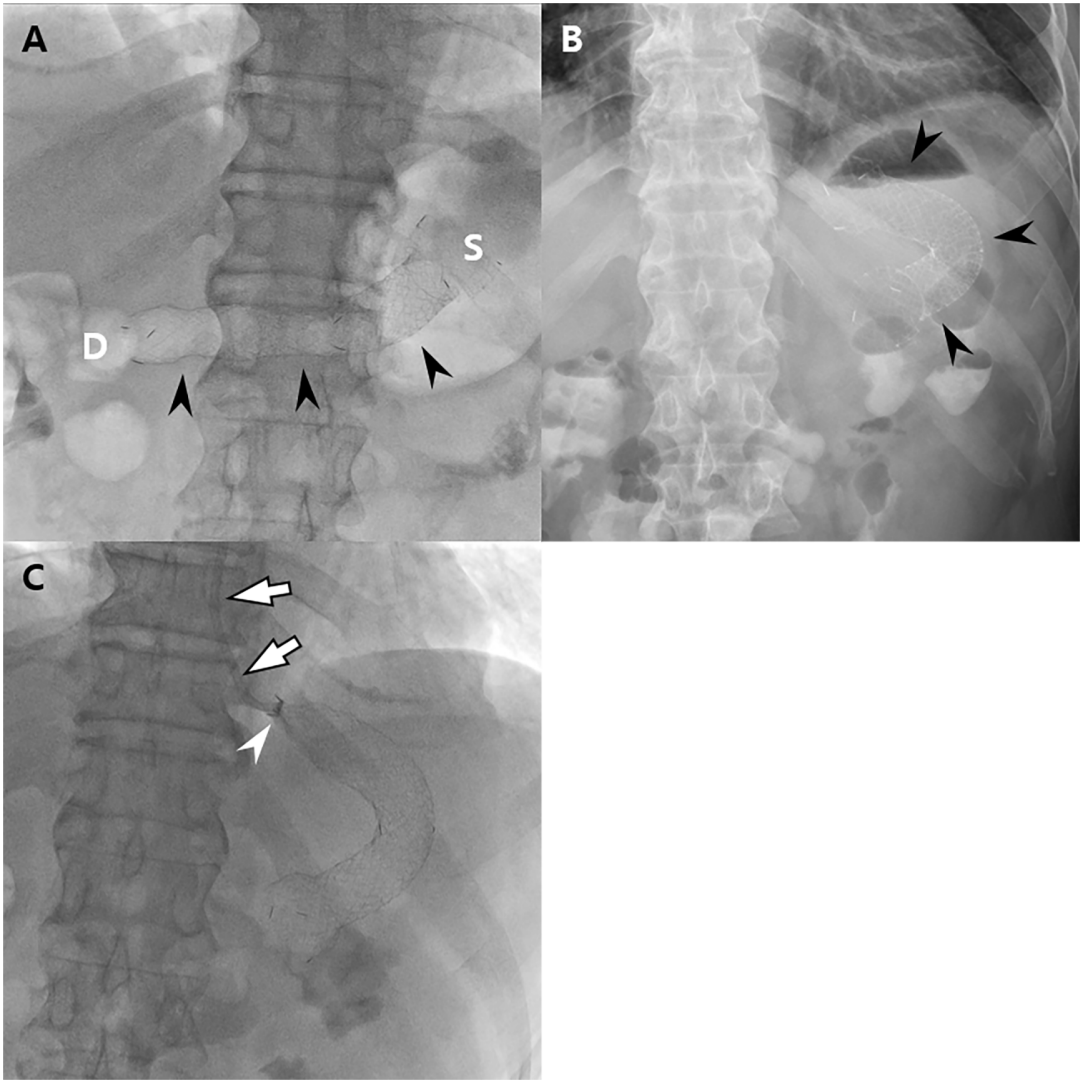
Images of a 56-year-old man with impaired pyloric function after laparoscopy-assisted pylorus-preserving gastrectomy (PPG). The patient underwent balloon dilatation and subsequent stent insertion 21 and 23 days after PPG, respectively. (A) On the scout image obtained after stent insertion, the stent (arrowheads) is well placed extending from the stomach (S) to the duodenum (D) with its center located at the pyloric canal. (B) On plain abdominal radiograph obtained after 1 day, the stent (arrowheads) has migrated proximally and is located within the remnant stomach. (C) Fluoroscopic guided stent removal was done using an angiographic catheter (arrows). Note the collapsed proximal end (arrowhead) of the stent.
